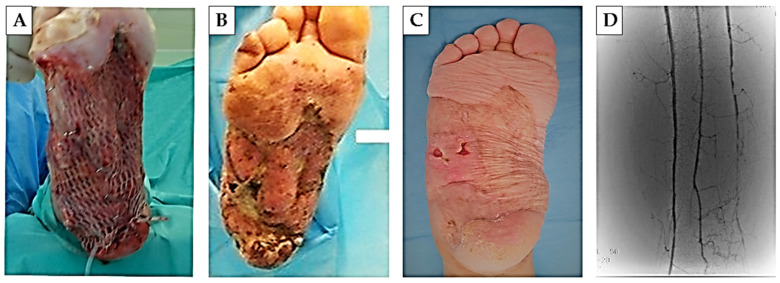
Wound healing progress. On the seventh day of treatment, an autologous skin graft measuring 80 cm^2^ was applied (A). Skin defect after four (B) and eight months (C) of therapy. The result of the angio-CT examination after eight months of treatment (D).
